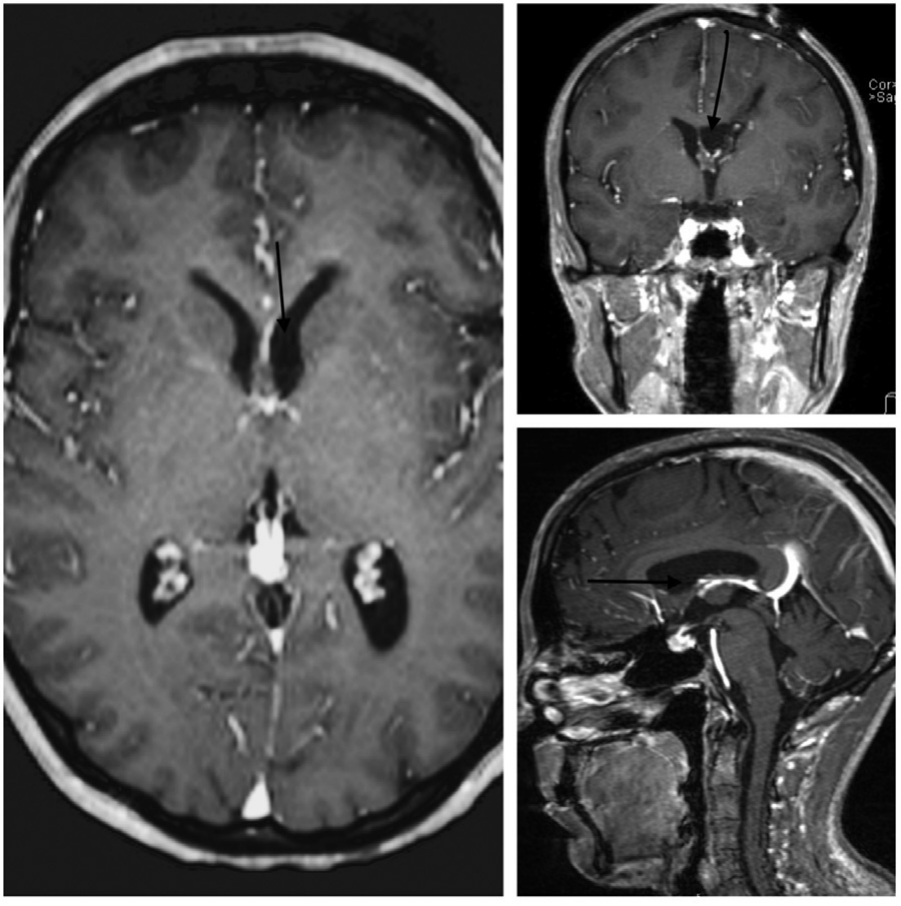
Post-operative brain MRI with contrast showing complete resection of the nodular enhancing lesion at the level of left foramen of monro without evidence of residual enhancement. Moreover, ventricular caliber improved considerably compared to pre-operative images.
